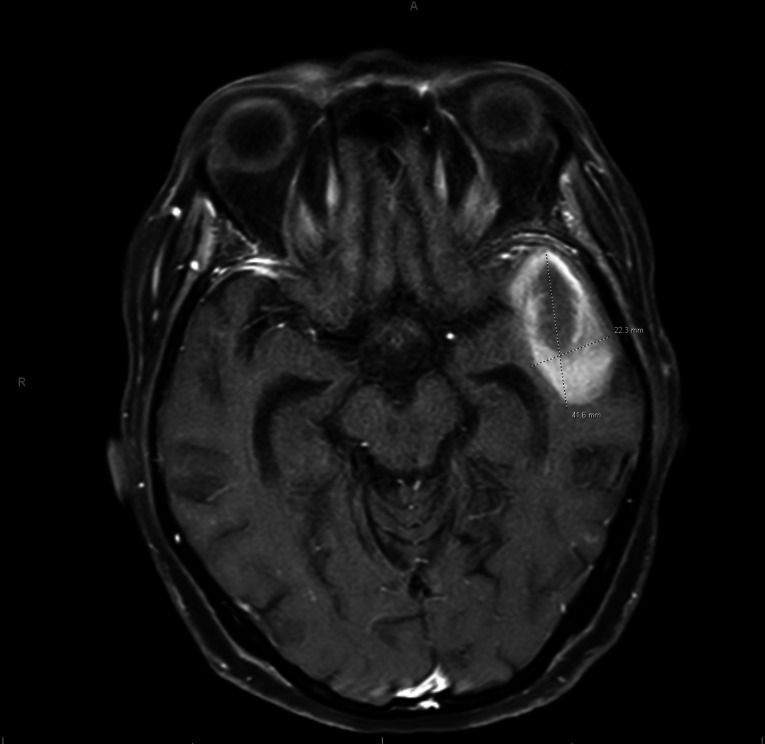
Magnetic resonance imaging of the head with and without IV contrast, showing complex cystic/proteinaceous structure without clear evidence of enhancing component or surrounding edema or mass effect, measuring about 4.2 × 2.2 cm in size, consistent with evolving subacute to chronic hematoma, neoplasm, or abscess with atypical features.
